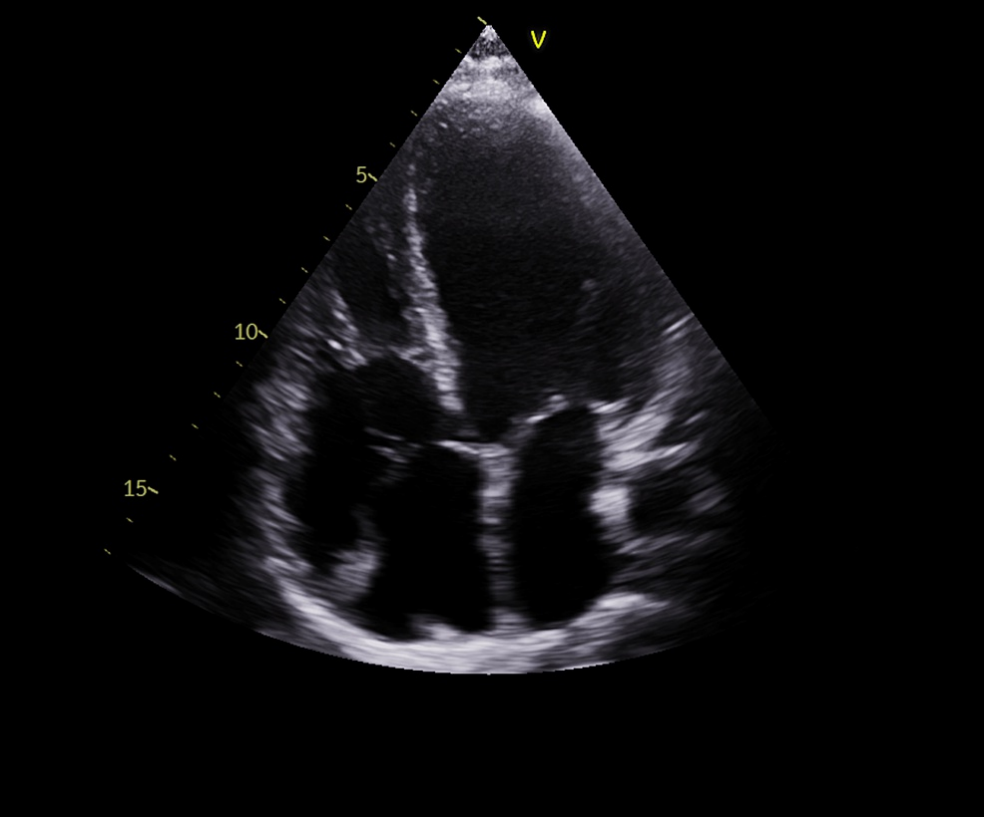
Apical four-chamber view on transthoracic echocardiogram demonstrating biatrial enlargement, global hypokinesis, and right ventricular dysfunction.
